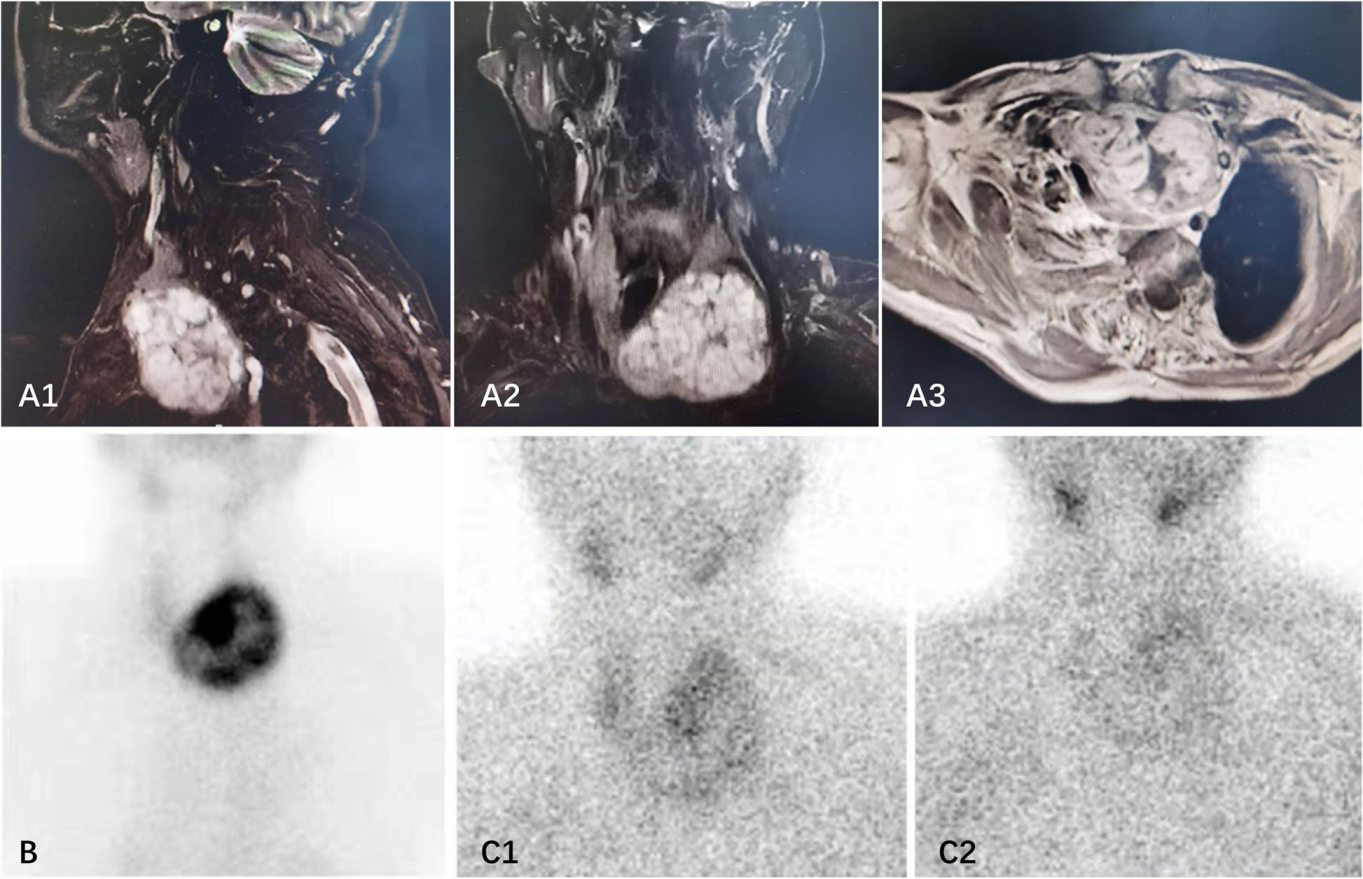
A Neck MRI showing a hyperdense lesion in the left lower thyroid gland, and trachea displacement to right side; B ^99m^TcO4 scintigraphy: A focus of increased uptake at the left thyroid pole, and multiple ‘hot’ thyroid nodules in the left and isthmus of thyroid gland, making the consideration of giant thyroid goiter; C 30 min after intravenous injection with 99MTC-MIBI showed abnormal appearance and left thyroid gland enlargement. 2 h later, there was a slightly hyperactive area in the left lower thyroid gland
